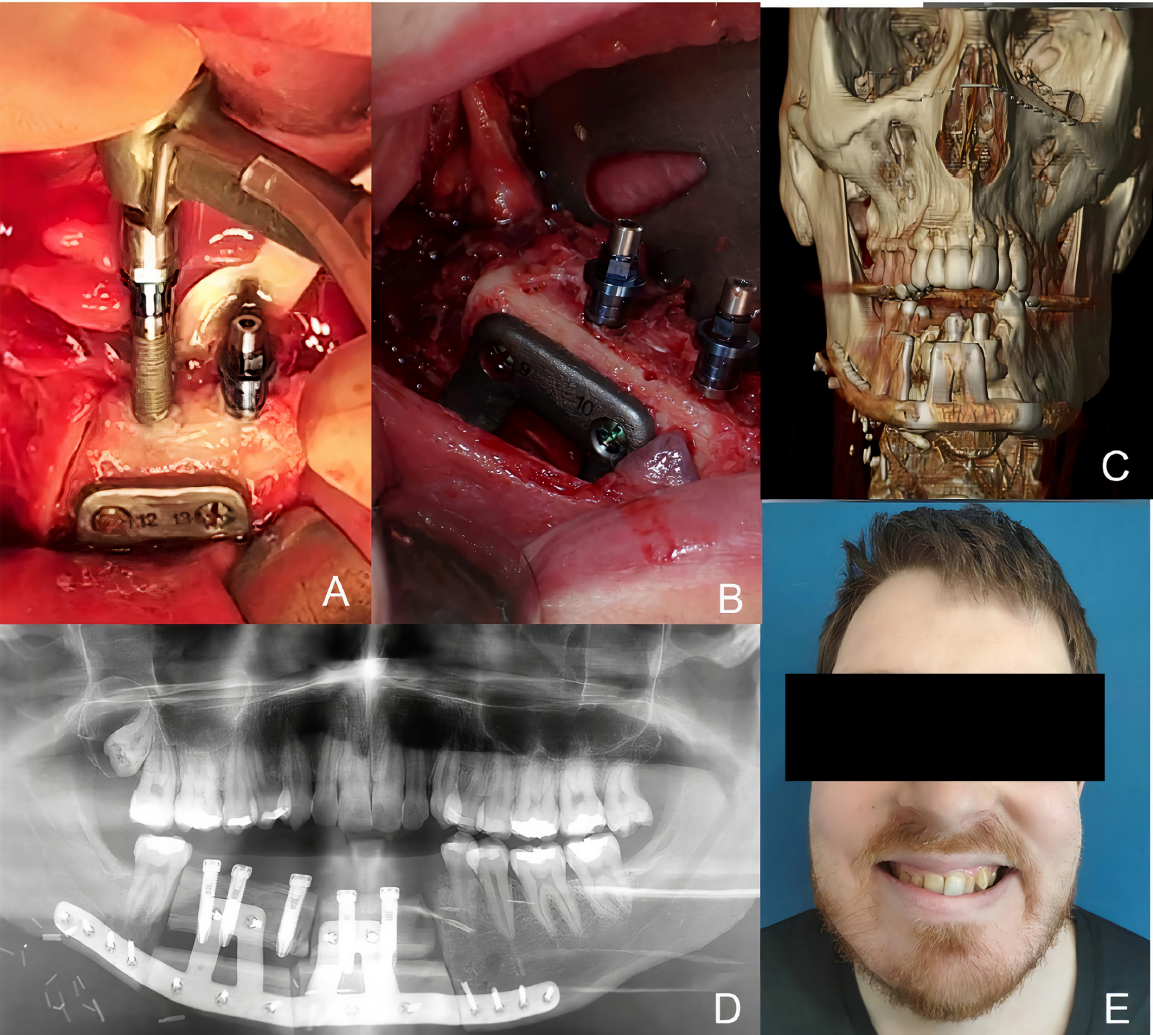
(A, B) Immediate implant placement between the osteosynthesis material as previously planned in the VSP. (C) Postoperative CT scan demonstrating the 3D reconstruction with the double-barrel free flap, the double-barrel customized plate and the immediate implants. (D) Panoramic radiograph after surgery with 3D reconstruction of the mandible. (E) Final aesthetic and functional result.
